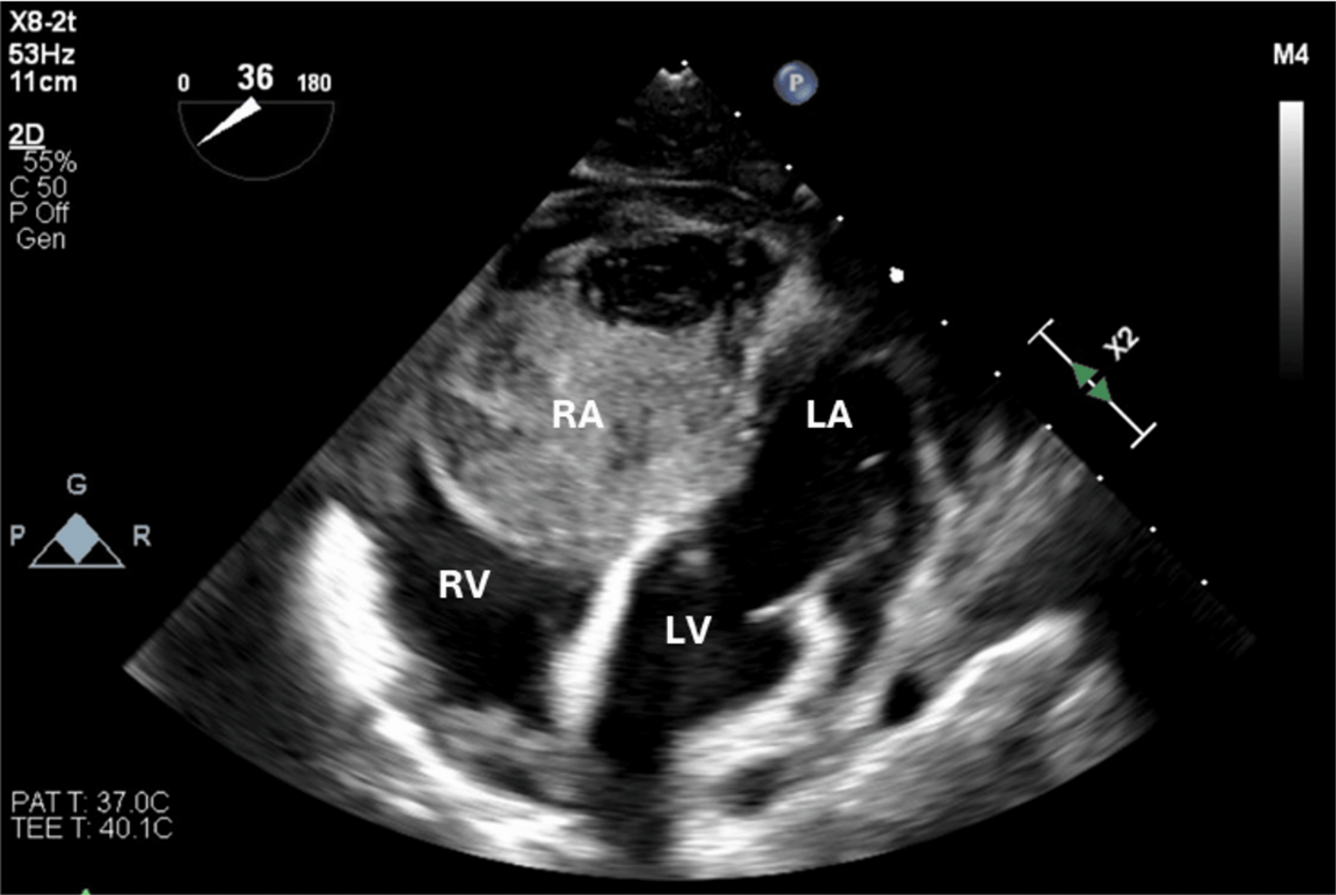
Transesophageal echocardiogram depicting the right atrial myxoma. RA: right atrium; RV: right ventricle; LA: left atrium; LV: left ventricle
